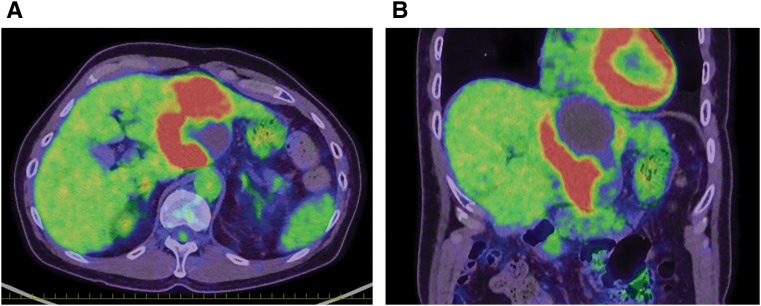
FDG PET findings for the tumor (A). Panel (A) shows axial FDG PET images of the tumor. High FDG uptake (SUVmax 15.96) is observed in the solid area. The tumor area without FDG uptake is suspected to be necrotic or hemorrhagic on the basis of imaging findings. No distant metastases are seen (B). Panel (B) shows coronal FDG PET images of the tumor. FDG, ^18^F-fluorodeoxyglucose
